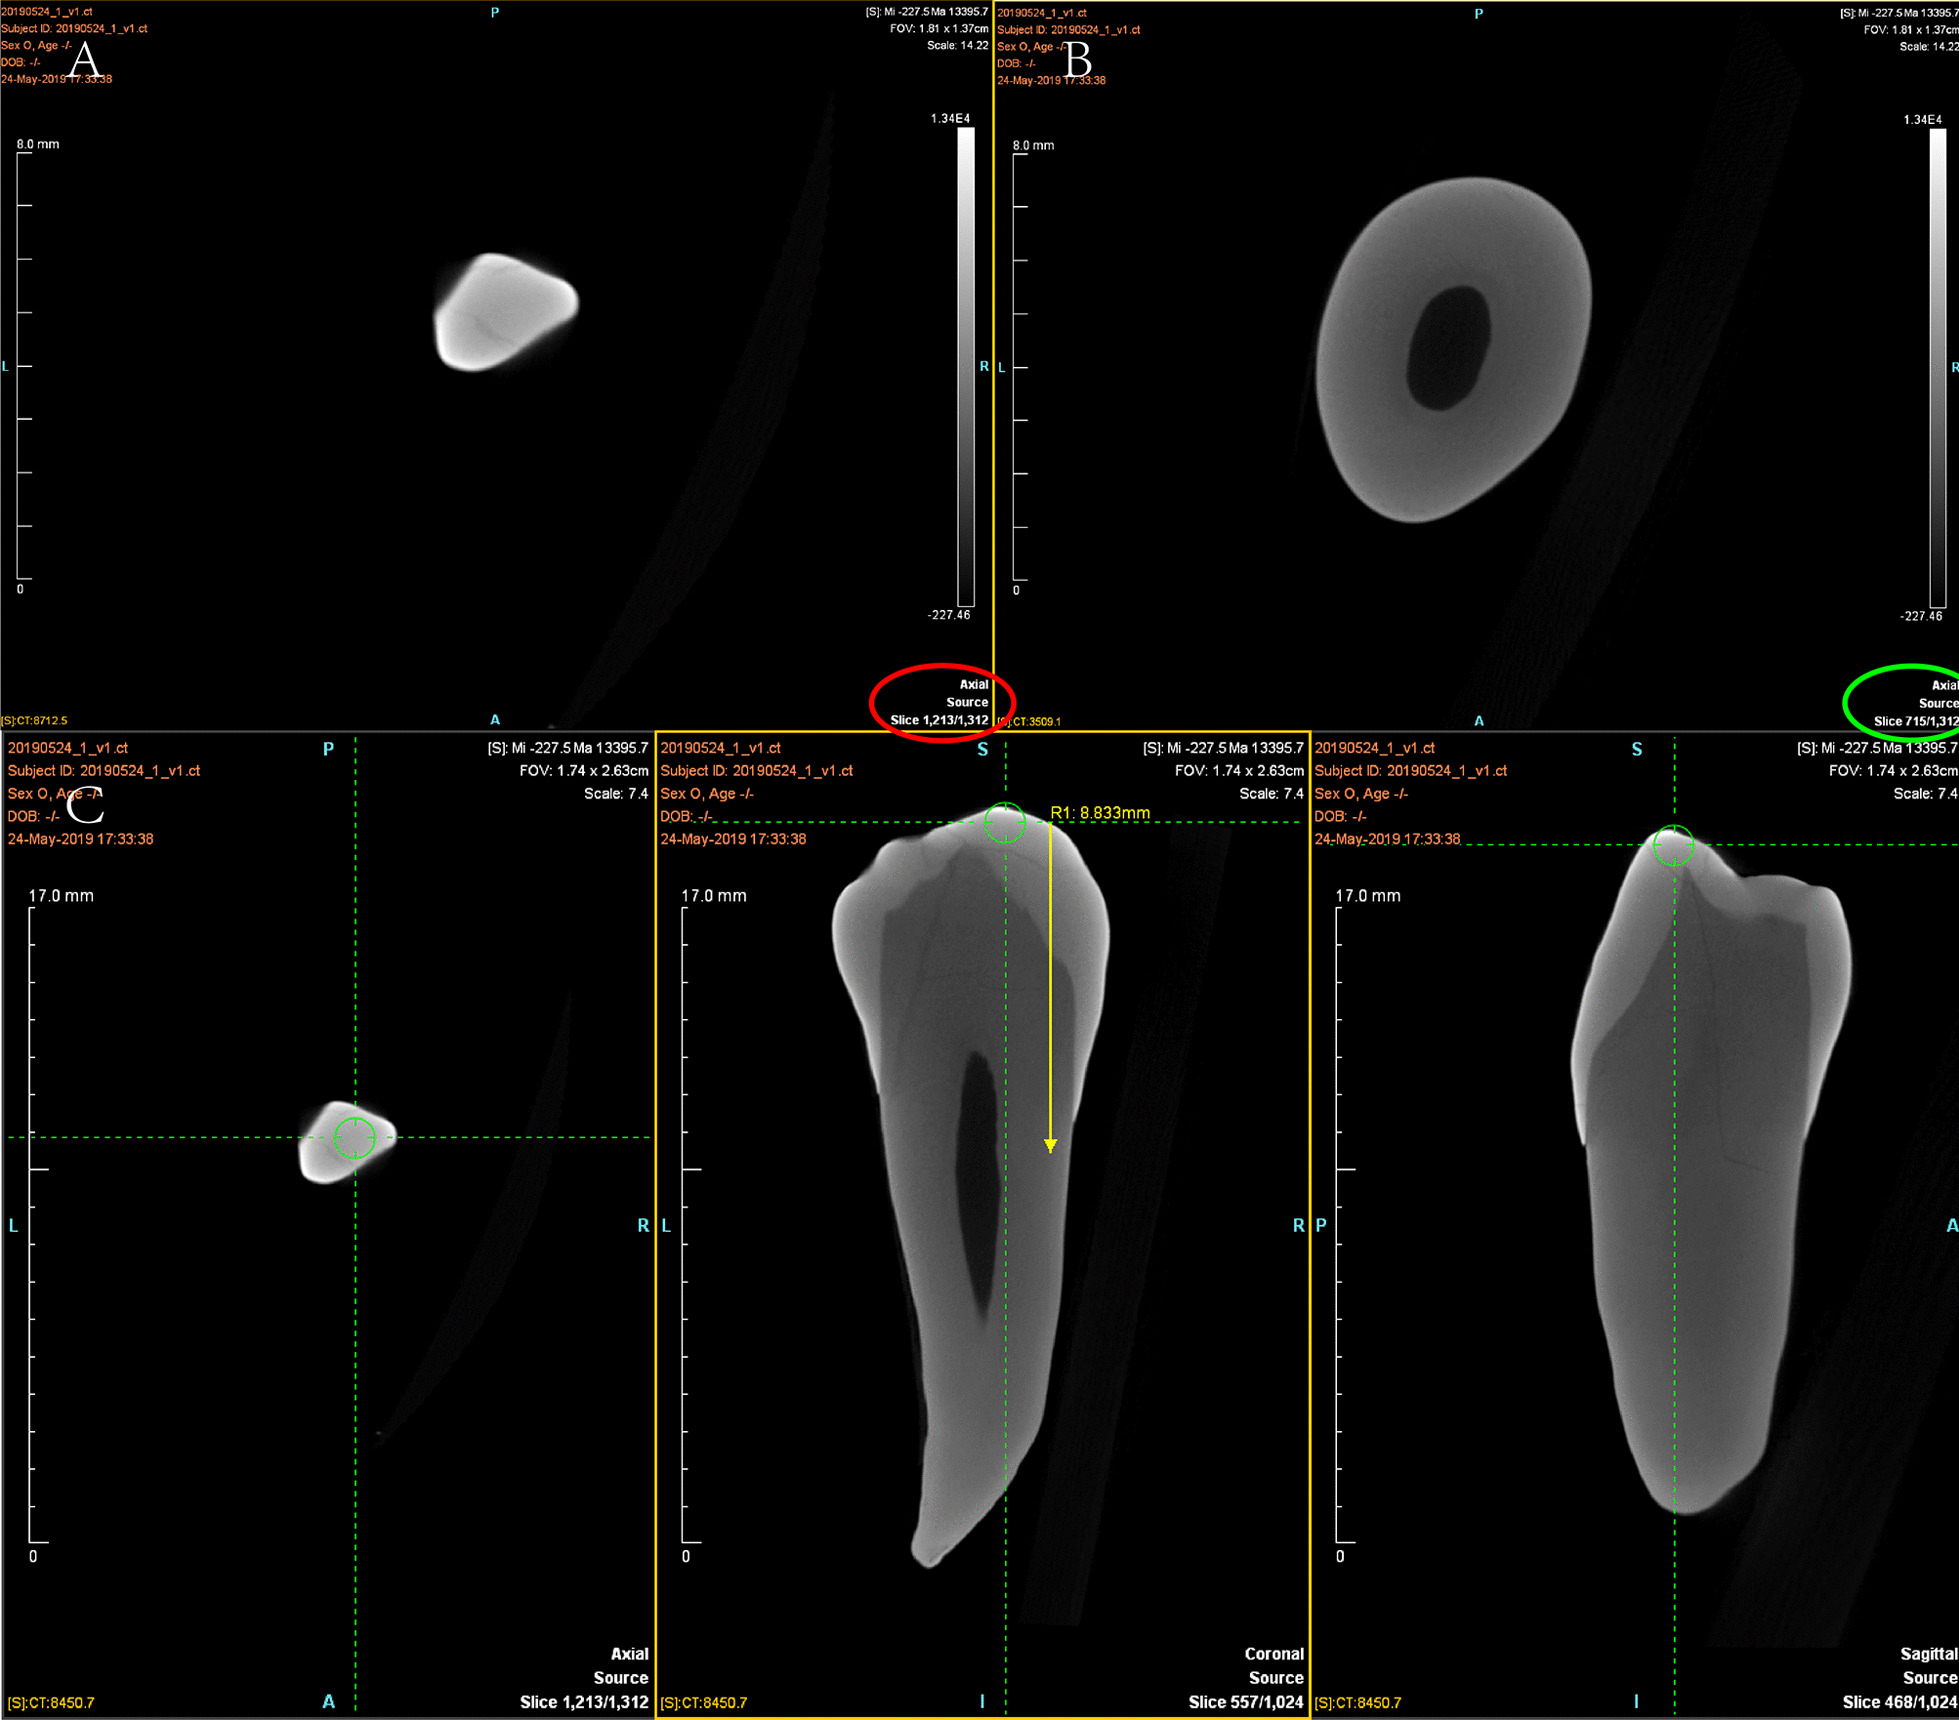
Measurement of the crack depth of a cracked tooth on micro-computed tomography. A Appearance of the crown crack on the horizontal plane at layer 1213 (indicated with a red circle); B complete disappearance of the root crack at layer 715 (indicated with a green circle); C the distance from layer 1213 to layer 715, as measured with the ruler tool (indicated with a yellow line), is the crack depth; in this case, the depth is 8.833 mm
